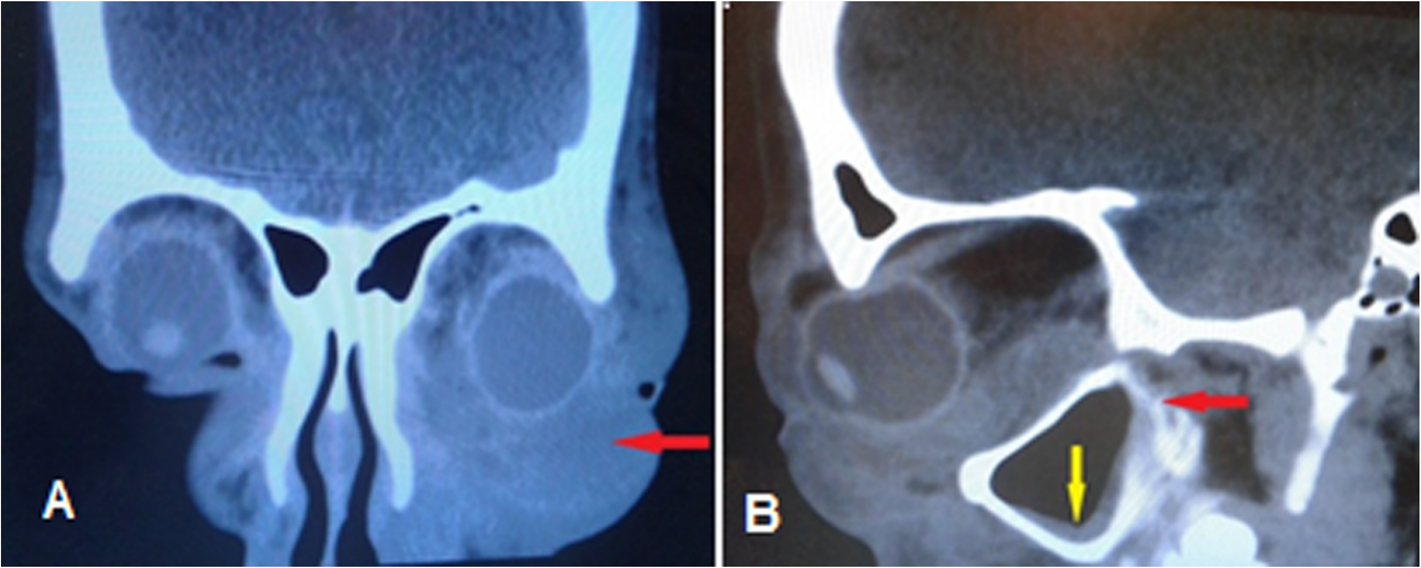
A 19 year-old diabetic patient with inferior orbital cellulitis secondary to dental abscess, a Orbital CT scan coronal view revealed inferior orbital abscess (red arrow), b axial view revealed mild mucosal thickening of maxillary sinus (yellow arrow) and retro-maxillary opacity (red arrow)
